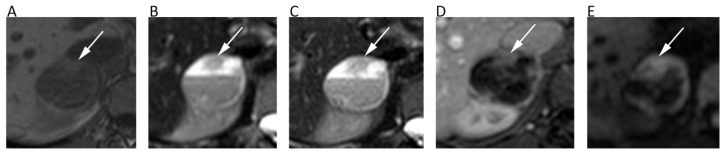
A 28-year-old male patient with pheochromocytoma of the right adrenal gland. Axial in-phase T1w (A), axial T2w (B), and axial fat-suppressed T2w (C) images show a right adrenal cystic lesion with fluid-fluid level. Axial post-contrast fat-suppressed 3D GRE T1w (D) and *b* = 600 DWI (E) allow one to better depict the solid component of the lesion consisting of the peripheral wall and the anterior nodular portion (arrows), with no restricted pattern of diffusion in central cystic part.
